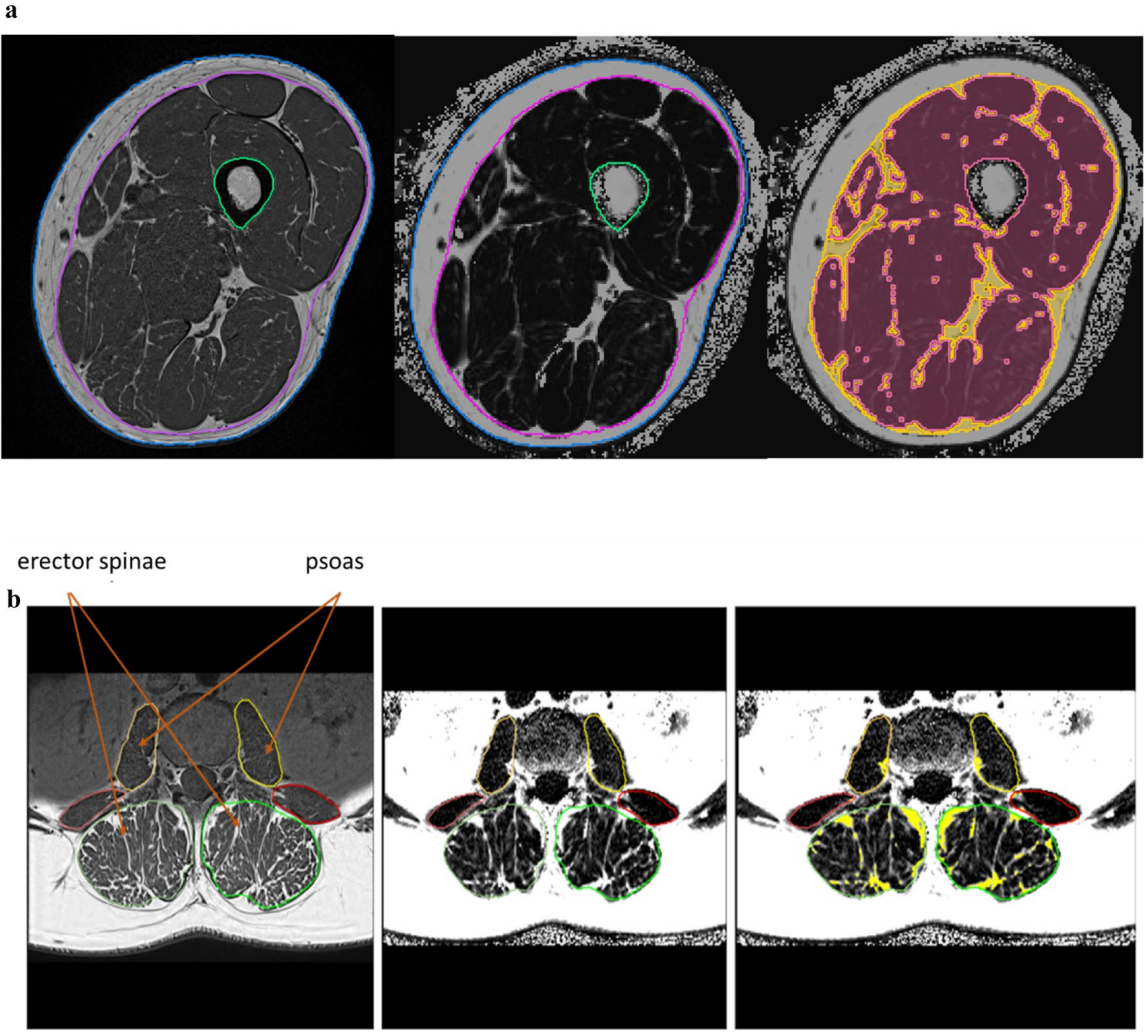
a MRI of the thigh. Left: T_1_ weighted sequence with segmented fascia lata; center: Dixon fat fraction image; right: separation of IMAT (yellow) and muscle tissue (red). b MRI of paraspinal muscles. Left: T_1_ weighted sequence with segmented muscles; center: Dixon fat fraction image; right: separation of IMAT (yellow) and muscle tissue (dark)
